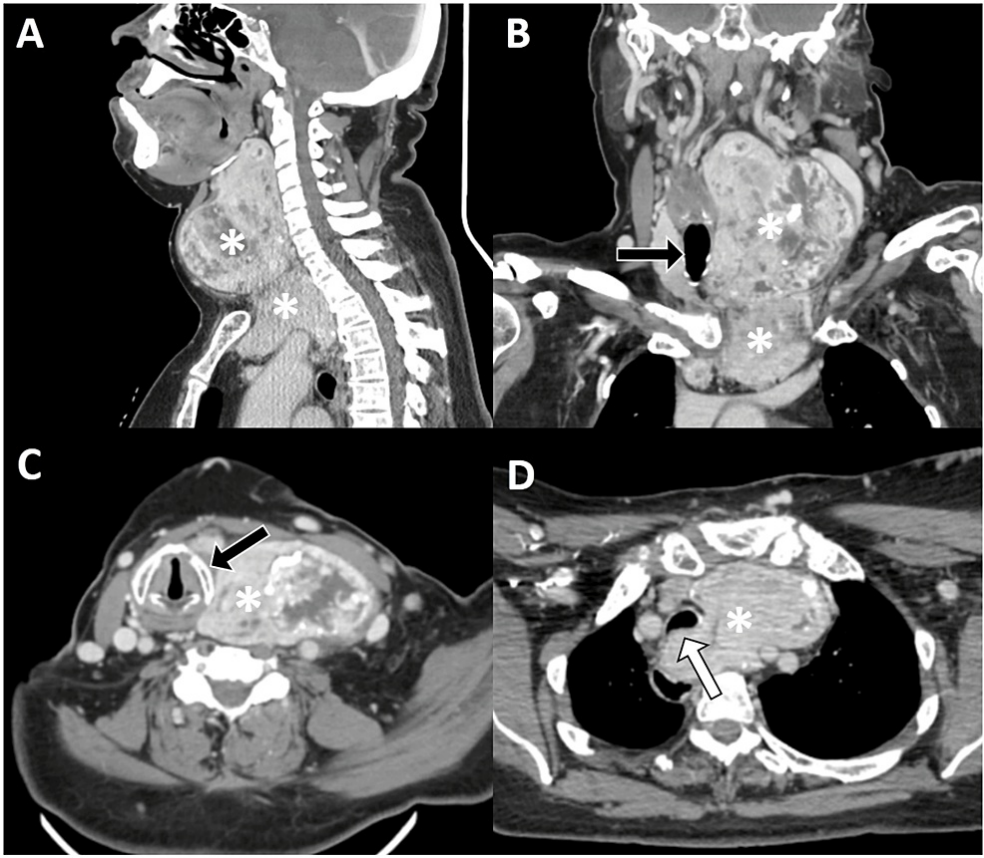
Preoperative CT scan. Saggital (A) and coronal (B) images showed a voluminous left cervical goiter with upper mediastinal extension (asterisks), measuring 10.2 x 6.8 x 15.3 cm (transverse x anteroposterior x longitudinal). Lateral right deviation of the trachea and larynx (black arrow). The axial cervical image (C) demonstrated a left goiter resulting in right deviation of the larynx (black arrow). The axial upper mediastinal image (D) demonstrated compression of the trachea (white arrow).
